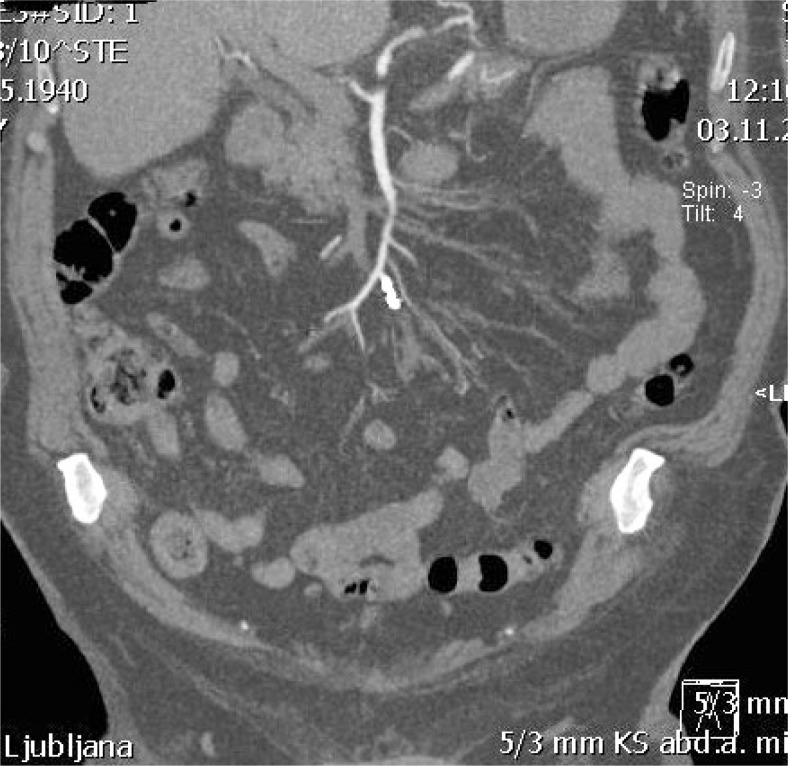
Coronal MIP reconstructions of CTA 45 months after the treatment, revealing patent SMA and branches. Coils permanently occluded ruptured branch.
